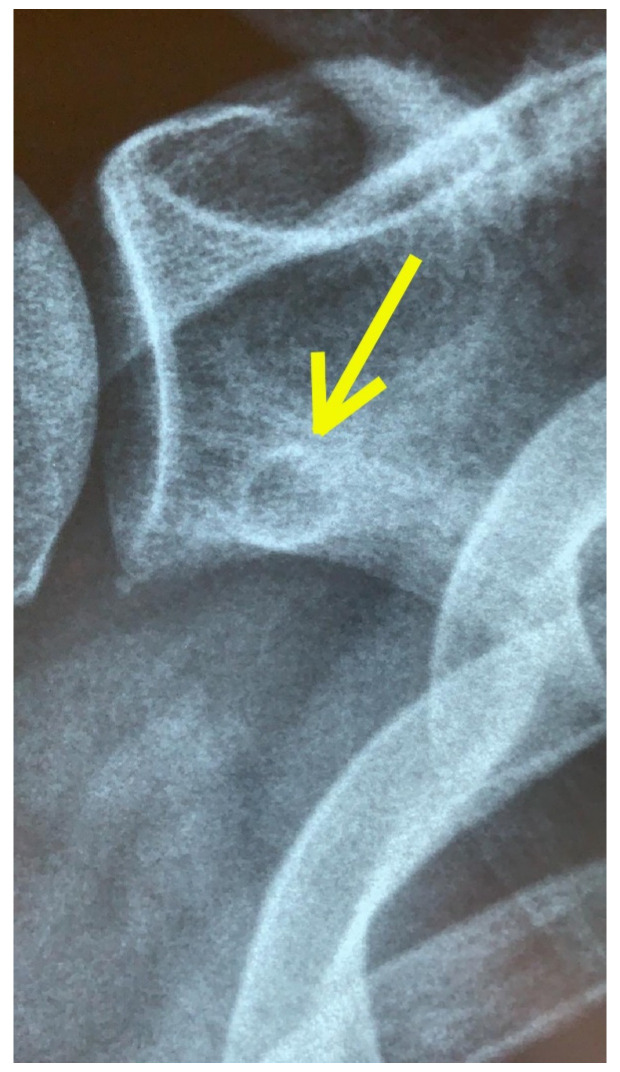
X-ray imaging. A small radiolucent formation is present on the lower portion of the scapular neck with hyperdense margins and an intact cortical rim of non-univocal interpretation (yellow arrow).
